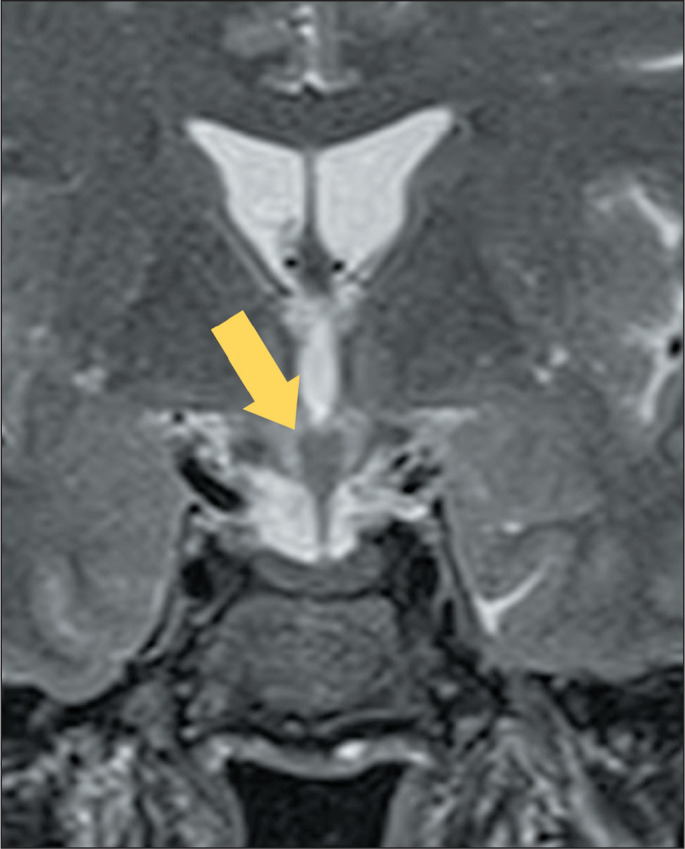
MRI of a patient with Erdheim-Chester disease. Coronal T2WI showing
